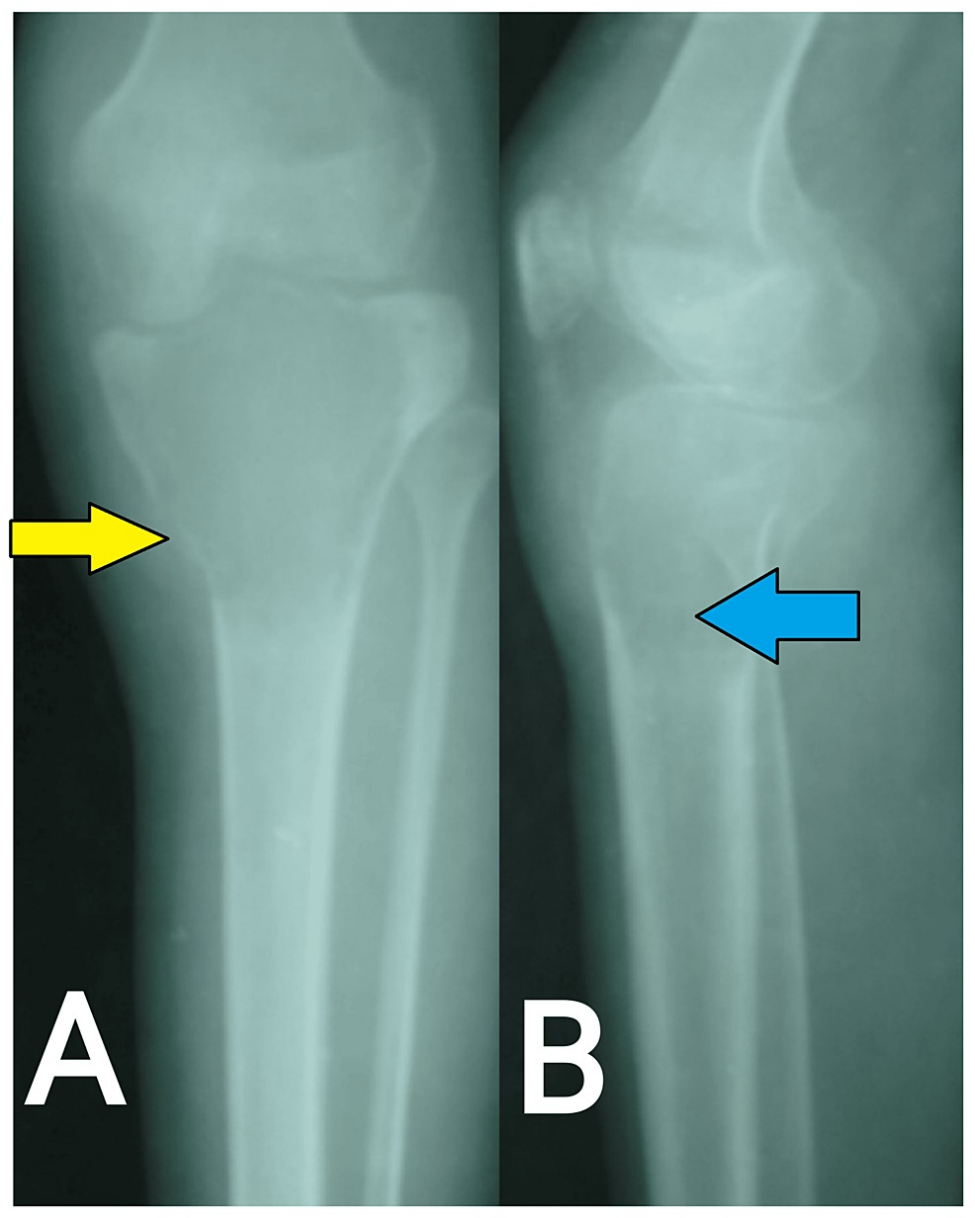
A plain radiograph of the right knee with the proximal tibia (anteroposterior and lateral views) displaying a lytic lesion over the proximal end of tibia with thinned-out cortices. (A) Anteroposterior view showing radiolucency and thinned-out medial cortex (yellow arrow); (B) Lateral view showing radiolucent area (blue arrow).
